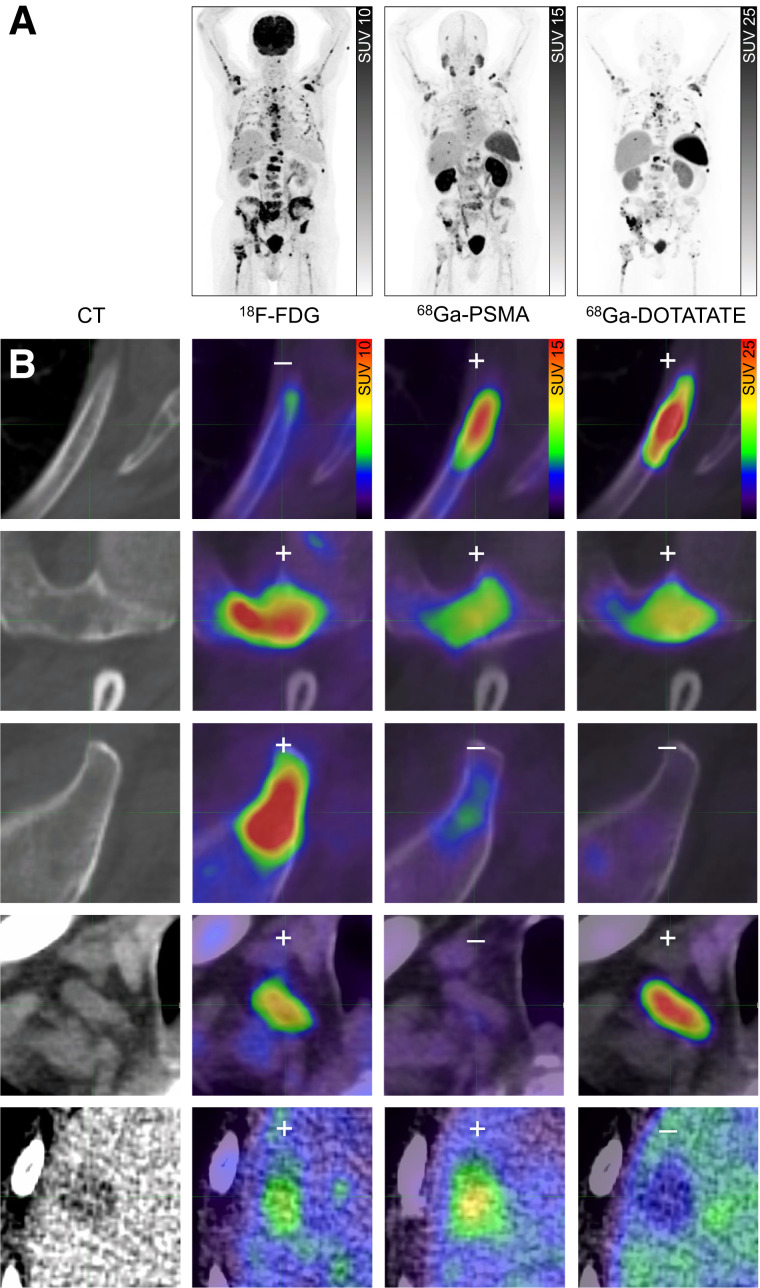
Patient with 5 different lesion phenotypes seen on triple-tracer PET/CT imaging. (A) Coregistered transaxial slices of whole-body maximum-intensity projection PET/CT showing multiple metastases with 3 different radiopharmaceuticals. (B) Discordant expression of 5 representative lesions: bone lesions of seventh left rib (first row) and left scapula (second row), lesions in left iliac (third row) and left supraclavicular (fourth row) lymph nodes, and lesion in right liver lobe (fifth row).
